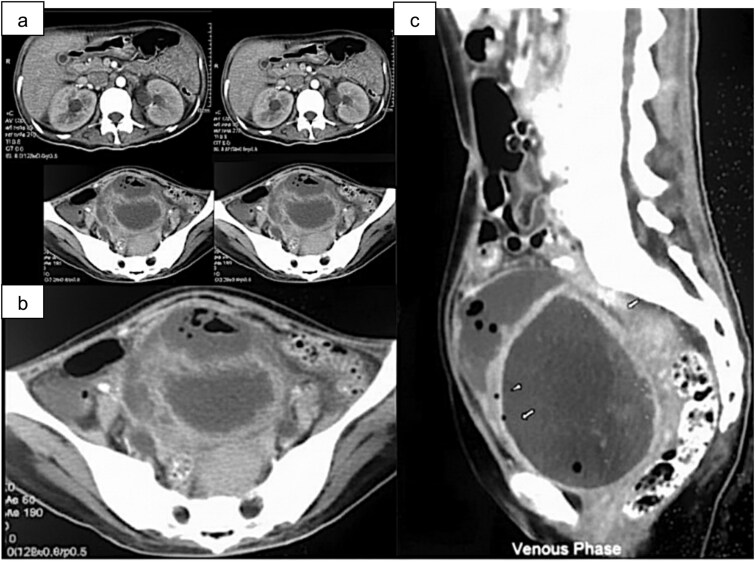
Contrast-enhanced abdominal CT. (a) Bilateral hydronephrosis. (b) Prevesical gas-fluid collection (short arrow). (c) Distended bladder exhibiting intramural (arrowhead) and intraluminal gas (long arrow).
